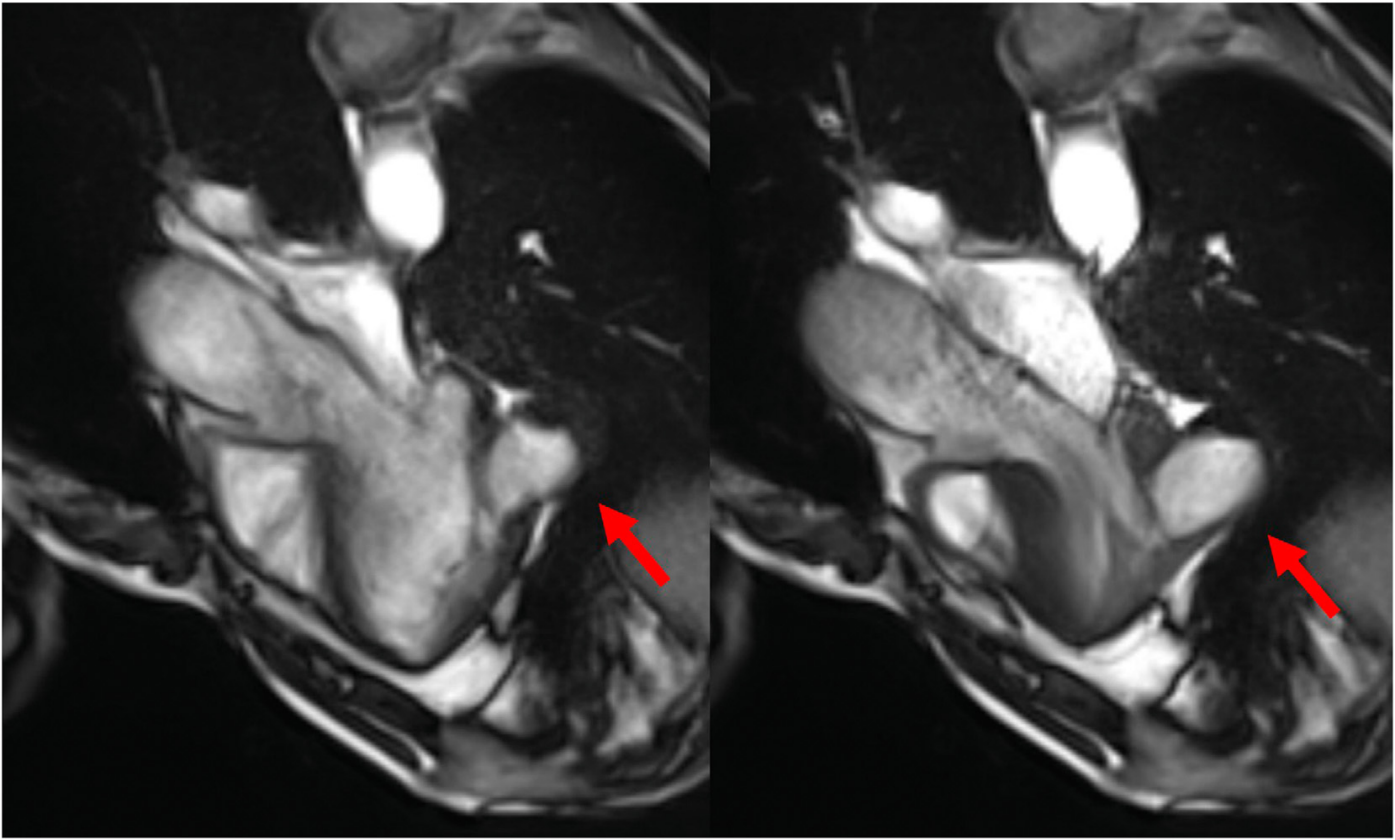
Cardiovascular magnetic resonance imaging, bSSFP cine sequence, oblique axial, long axis, 3-chamber diastolic (*left*) and systolic (*right*) display, demonstrates normal global LV systolic function and the large LVP (*arrow*).
